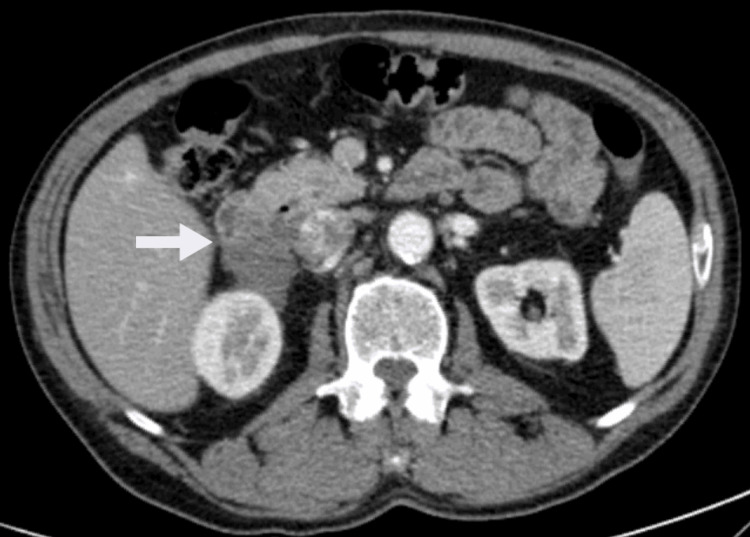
Reassessment CT scan after 12 months of neoadjuvant imatinib showing a decrease in tumor size CT: computed tomography
